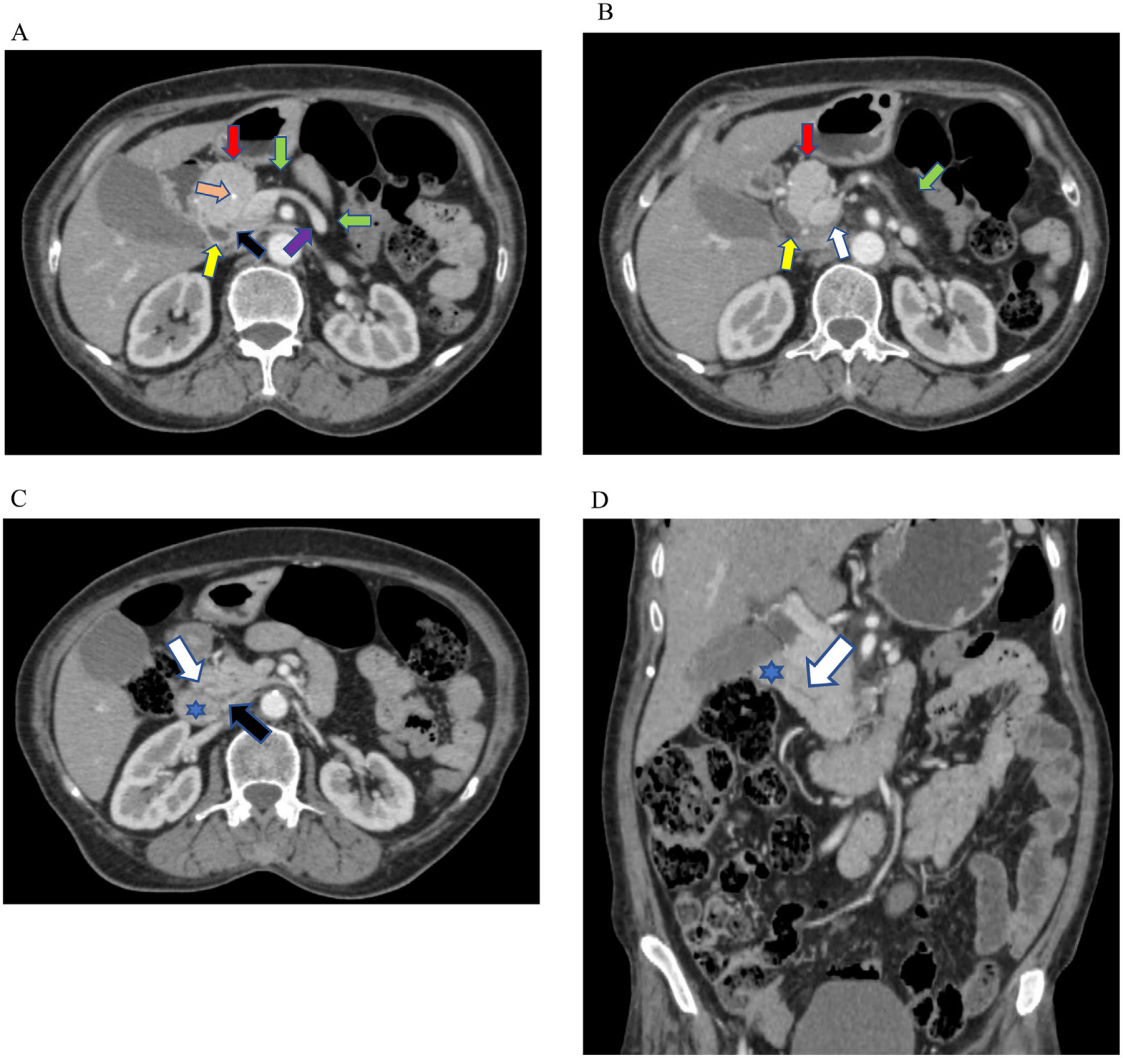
(A-D) Contrast-enhanced selected axial and coronal CT images of the abdomen revealing the uncinate process and part of the head of the pancreas. (red arrow). The rest of the pancreas appears absent, instead occupied by the retroperitoneal fat (green arrows) anterior to the splenic vein (purple arrow). The duct of Santorini accessing the minor papilla (white arrow) and the duct of Wirsung (black arrow) communicating with the major duodenal papilla (blue arrow) of the duodenum (blue asterisk). Foci of dense calcifications present within the ventral pancreas (orange arrow). The distal common bile duct (yellow arrow) and portal vein (white arrow) are noted.
